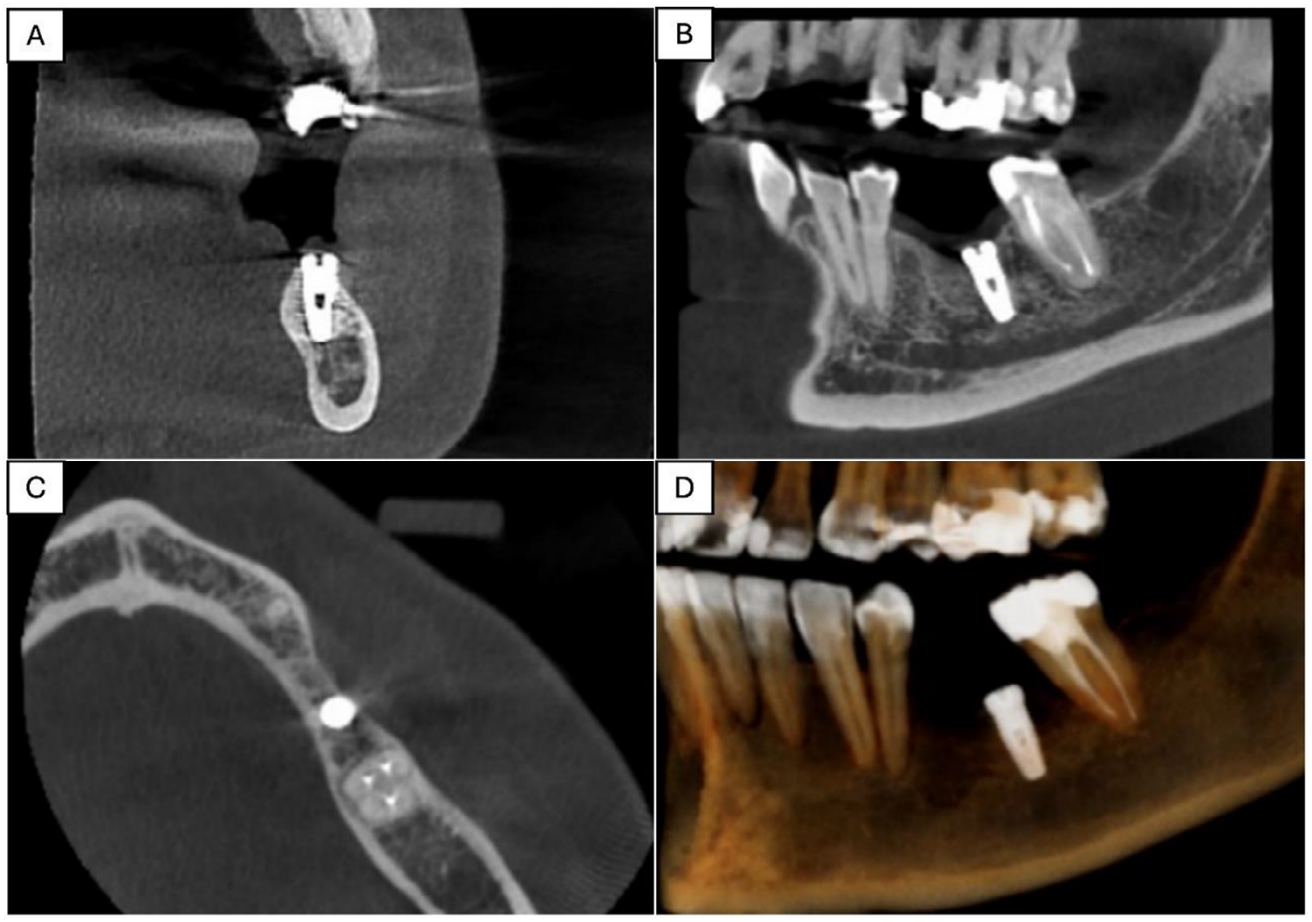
Cone beam computed tomography (CBCT) dental implant scan: (A) Coronal plane; (B) Sagittal plane; (C) Axial plane; and (D) 3D reformatting.
